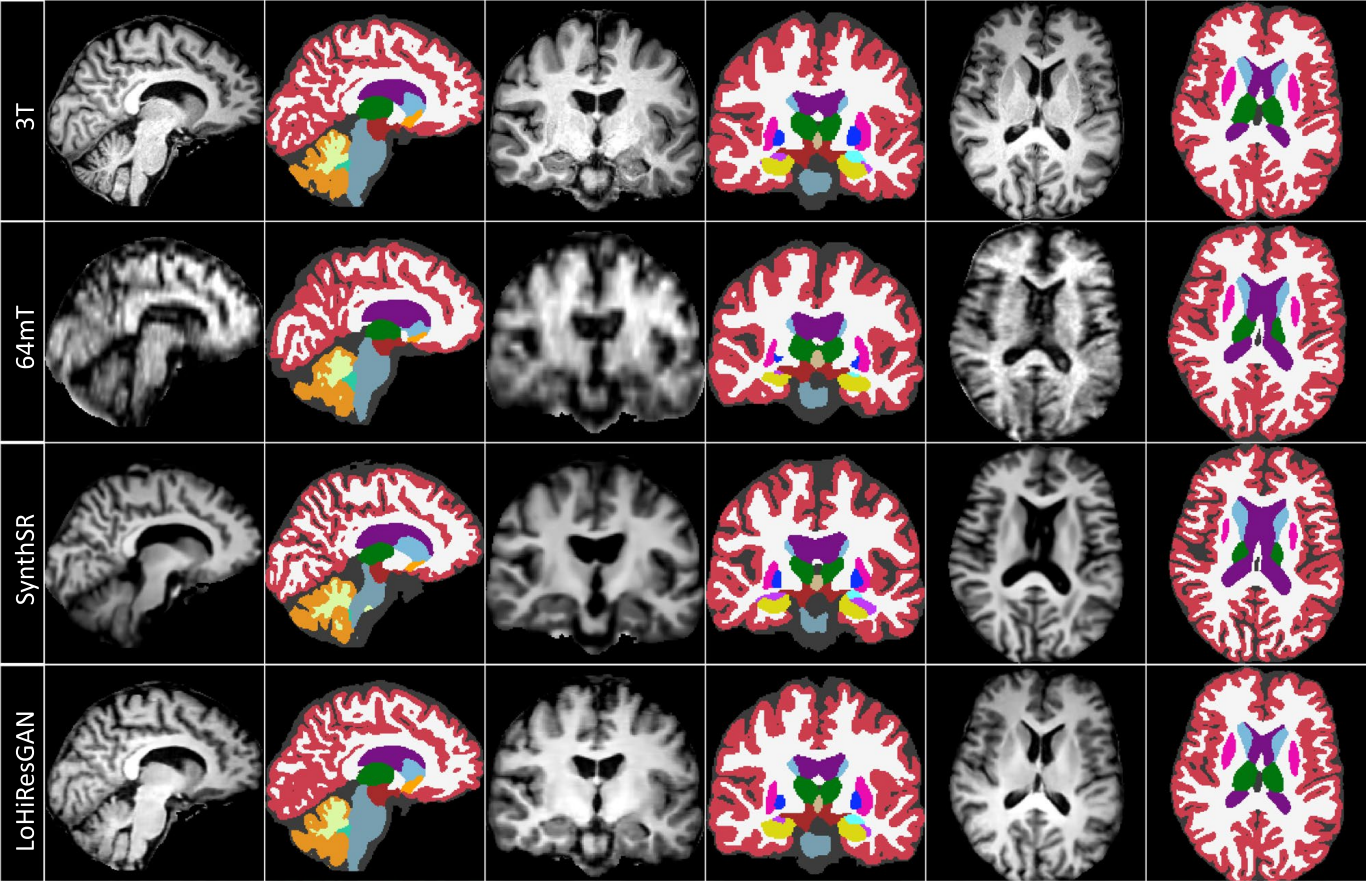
Brain regions visualised using orthogonal views (T1-weighted): Sagittal, Coronal, and Axial for 3T (first row), 64mT (second row), SynthSR (third row), and LoHiResGAN (fourth row).
